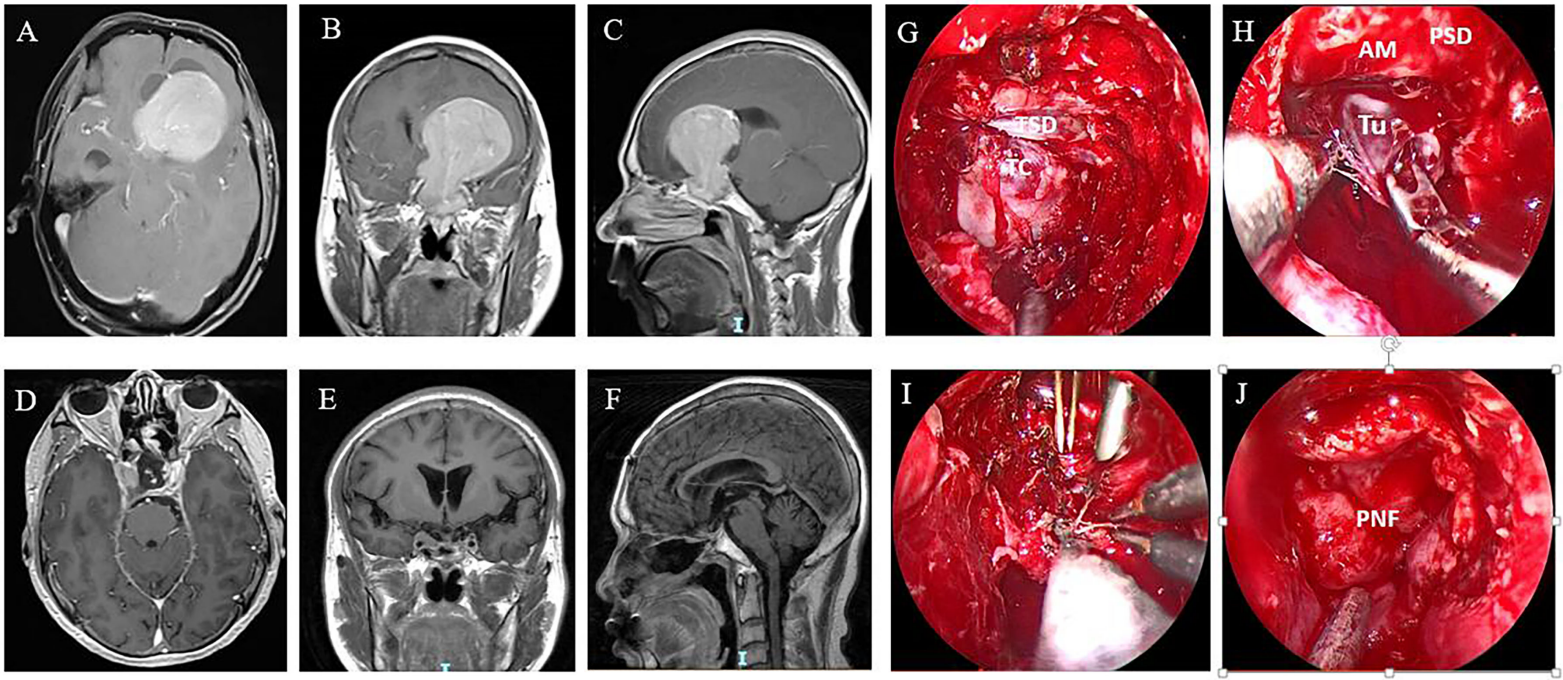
A GPA in a 46-year-old male patient. Preoperative axial (A), coronal (B), and sagittal (C) MR images show the tumor extending into the left lateral ventricle. The patient underwent ETS and a combined left frontal craniotomy via a transcortical approach. Postoperative MR images showing that the tumor was gross totally resected (D–F). (G–J) Intraoperative images of the surgical stages. (G) Endoscopic view after sellar opening. The dura was quite thin and adhered closely to the tumor capsule. (H) The suprasellar part of the tumor did not descend after intrasellar tumor resection and needed to be mechanically delivered from the cranial path. (I) The tumor was removed sufficiently with ETS and simultaneous TCS. (J) The skull base defect was reconstructed with an autologous fat graft, a pedicled nasoseptal flap, and oxidized cellulose with fibrin glue. TSD, tuberculum sella dura; TC, tumor capsule; Tu, tumor; AM, arachnoid membrane; PSD, planum sphenoidale dura; PNF, pedicled nasoseptal flap.
